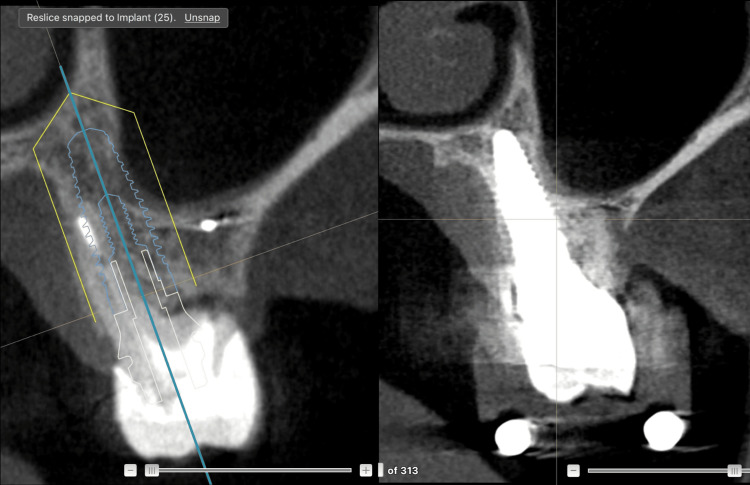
Comparison between the planned and postoperative CBCT images, showing the precise final implant position achieved with dynamic navigation CBCT: cone-beam computed tomography.
